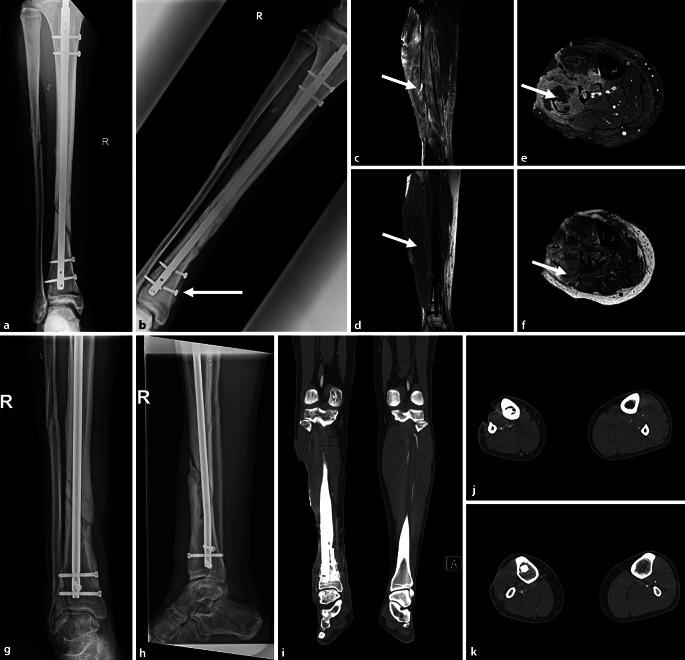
Röntgen-, Computertomograpie(CT)- und Magnetresonanztomographie(MRT)-Bilder des rechten Unterschenkels. a Nichtgeheilte Fraktur und Zustand nach Marknagelung 2 Monate nach Trauma, b Zustand der Fraktur 8 Monate nach dem Unfall mit gebrochenen Verriegelungsschrauben (*weißer Pfeil*) und der Pseudoarthrose. c–f MRT-Untersuchungsergebnisse des akuten Zustands nach Kompartmentsyndrom der Fibularisloge einen Monat nach Trauma mit Weichteildefekt und Atrophie sowie ödematöser Auftreibung der gesamten Flexoren- und Fibularisloge. Die *weißen Pfeile* weisen auf die Fibularisloge mit den Läsionen. g, h Knöcherne Konsolidierung, mit ventralseitigem Spalt sowie teilweiser Brückenbildung 2 Jahre nach dem Trauma. i–k Präoperative lokale Gefäßsituation in der CT-Angiographie ein Jahr nach dem Trauma
